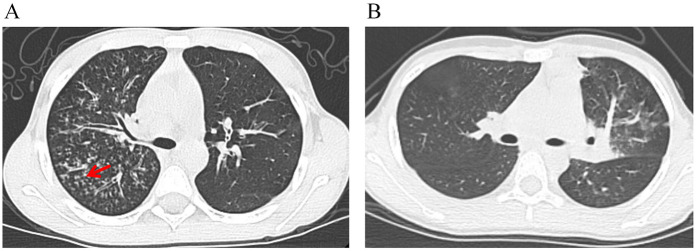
The risk of developing MUMPP was calculated for two pediatric patients using a nomogram. (A) A chest CT image from a boy with MPP shows the tree-in-bud sign (red arrow). His laboratory findings were as follows: NEUT-value, 10.22 × 10⁹/L; LYMPH-value, 1.98 × 10⁹/L; CK, 257 U/L; PLR, 267. The total nomogram score was approximately 158 points, corresponding to a probability of developing MUMPP of greater than 90%. (B) A chest CT image from a girl with MPP shows no tree-in-bud sign. Her laboratory findings were as follows: NEUT-value, 6.42 × 10⁹/L; LYMPH-value, 2.76 × 10⁹/L; CK, 197 U/L; PLR, 136. The total nomogram score was approximately 111 points, corresponding to a probability of developing MUMPP of less than 20%. NEUT-value, neutrophil count; LYMPH-value, lymphocyte count; CK, creatine kinase; PLR, platelet-to-lymphocyte ratio.
